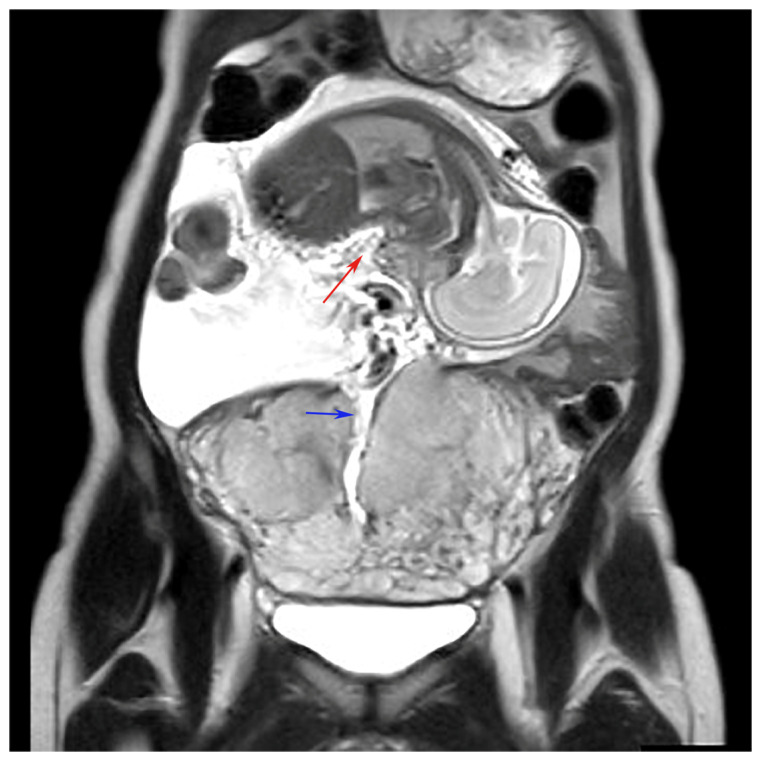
Pelvic MRI (coronal) showing that the muscular layer of the uterine fundus was ruptured and the fetus had completely prolapsed into the abdominal cavity. The fetus is indicated by the red arrow, and the wound in the uterus is indicated by the blue arrow.
